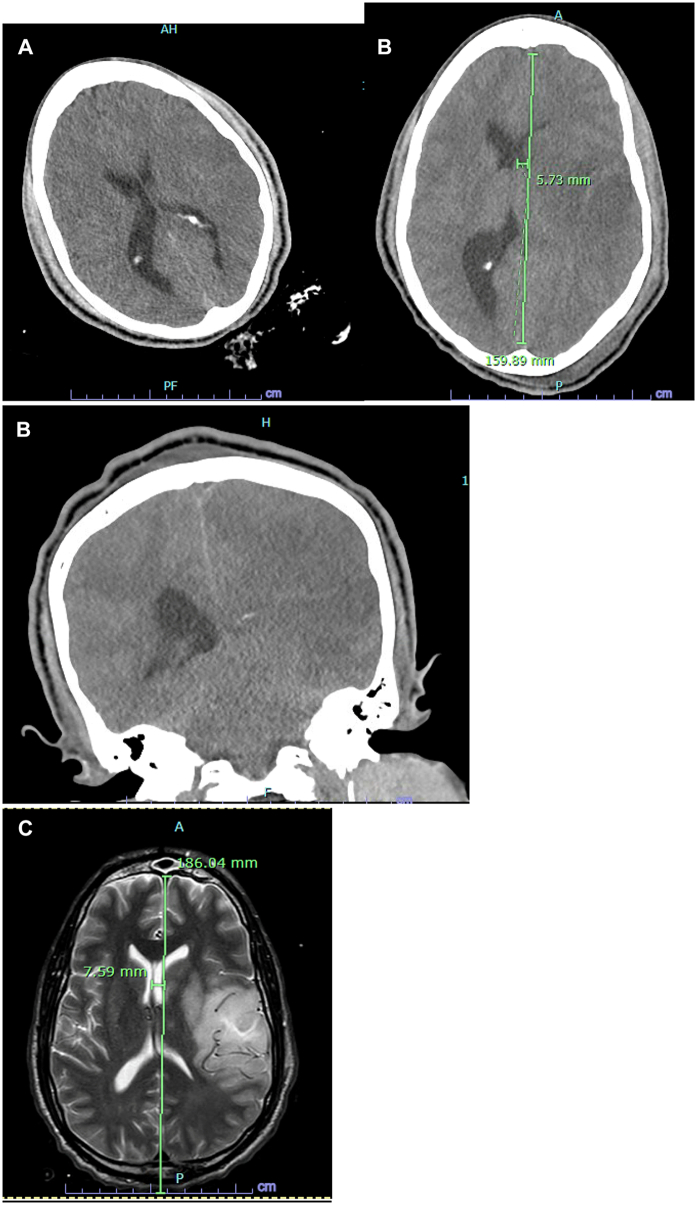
A: CT Head on the hospital/post-operative day 0 concerning loss of gray-white matter differentiation involving the left temporal lobe concerning the left middle cerebral artery (MCA) infarct. B: CT Head on hospital day 3 showing increasing midline shift and increasing ventricular dilation of the right lateral ventricle with large evolving left MCA infarct. C: MRI on hospital day 5 showing large left MCA acute infarct with edema and 8 mm of left to right midline shift
